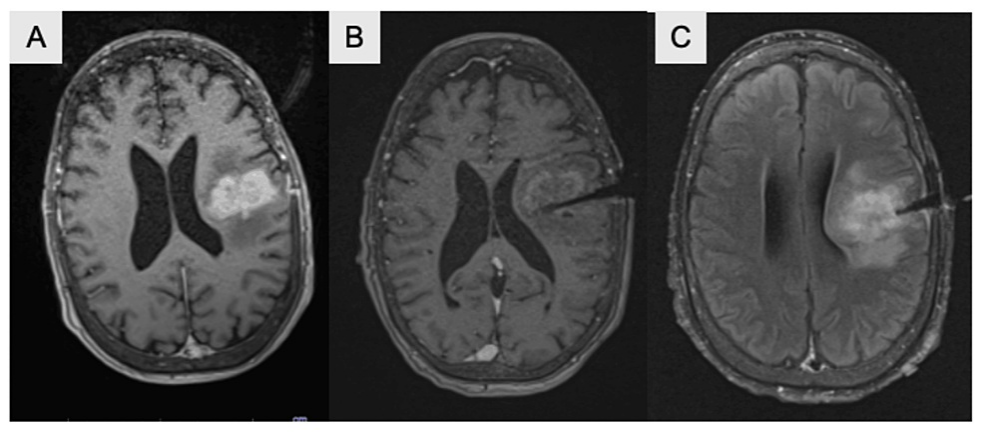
Post-ablation of MRI. T1-weighted MRI with contrast in the axial plane before (A) and after (B) ablation showing ablated region with reduced enhancement compared to preoperative films. (C) T2-weighted FLAIR MRI in the axial plane post-LITT showing an ablated region with increased signal. LITT: laser interstitial thermal therapy; FLAIR: fluid-attenuated inversion recovery.
